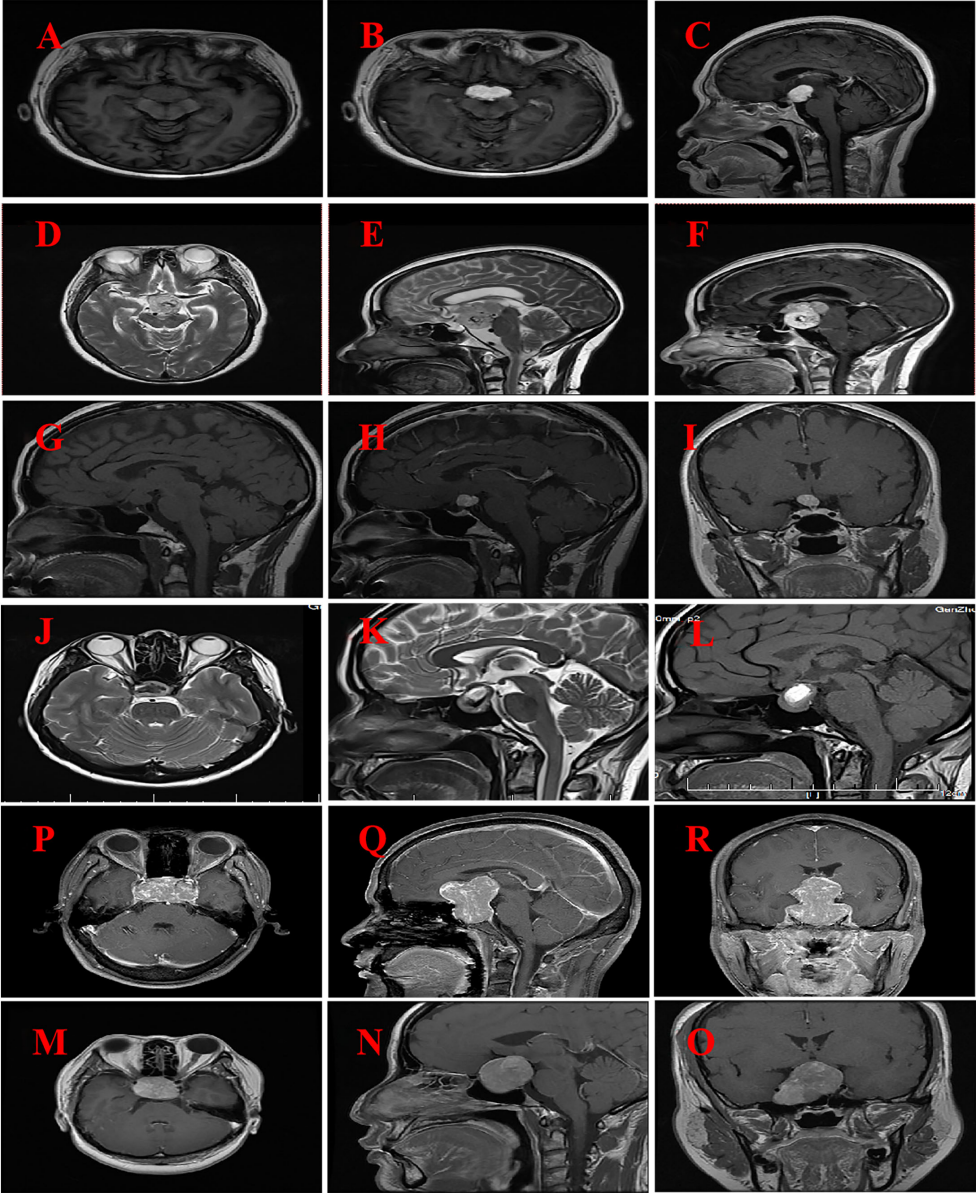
MRI imaging for six patients with GCT of the neurohypophysis. (A–C) The imaging reveals a well-defined, rounded lesion in the suprasellar region, characterized by a slightly prolonged T1 signal. Post-contrast enhancement demonstrates uniform and pronounced enhancement of the lesion, which appears to be in close proximity to the optic chiasm. (Case No. 1). (D–F) There are irregular lesions in the supratentorial region, tri-cerebral ventricles, and anterior pontine pools, characterized by slightly prolonged T2 signals and distinct borders. The enhancement scan reveals uneven and significant enhancement, closely associated with compression of the left thalamus and brainstem. (Case No. 2). (G–I) A rounded lesion located in the suprasellar region exhibited iso-intensity on T1-weighted imaging (T1WI), with well-defined margins. Post-contrast imaging demonstrated significant homogeneous enhancement of the lesion, resulting in compression and elevation of the optic chiasm. (Case No. 3). (J–L) Irregular lesions are observed in the sellar and suprasellar regions, characterized by short T2-weighted signal intensities and well-defined margins. Post-contrast imaging reveals heterogeneous enhancement of the lesions, with evidence of compression and elevation of the optic chiasm. (Case No. 4). (M–O) Solid lesions were observed in the saddle and suprasellar regions, exhibiting mild to moderate enhancement upon contrast-enhanced imaging. These lesions demonstrated bilateral invasion of the cavernous sinuses, encirclement of the internal carotid arteries, and upward extension into the suprasellar cisterns and third ventricles. Additionally, there was compression of the pituitary stalks and optic chiasm. (Case No. 5).(P–R) Solid lesions are observed both within and on the saddle. Upon enhanced imaging, these lesions exhibit moderate to significant enhancement, characterized by heterogeneous enhancement patterns. The lesions extend into the cavernous sinuses bilaterally, resulting in compression of the pituitary stalk and optic chiasm. (Case No. 6).
